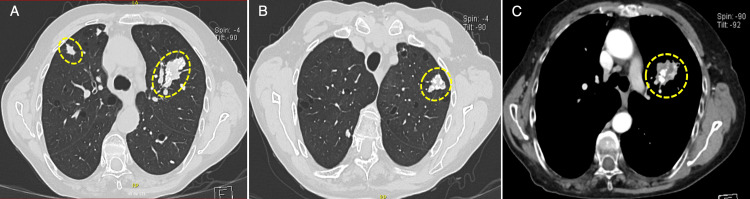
High-resolution computed tomography of the chest demonstrating pulmonary cysts and nodules (A) Axial non-contrast lung-window image shows numerous thin-walled pulmonary cysts (approximately 1-30 mm) with basal predominance (yellow dashed circles). (B) Axial contrast-enhanced mediastinal-window image again highlights the diffuse cystic changes (yellow dashed circles). (C) Axial contrast-enhanced mediastinal-window image shows two solid, heterogeneous pulmonary nodules with internal calcified foci and heterogeneous enhancement in the left apical and apicoposterior segments (yellow dashed circle).
